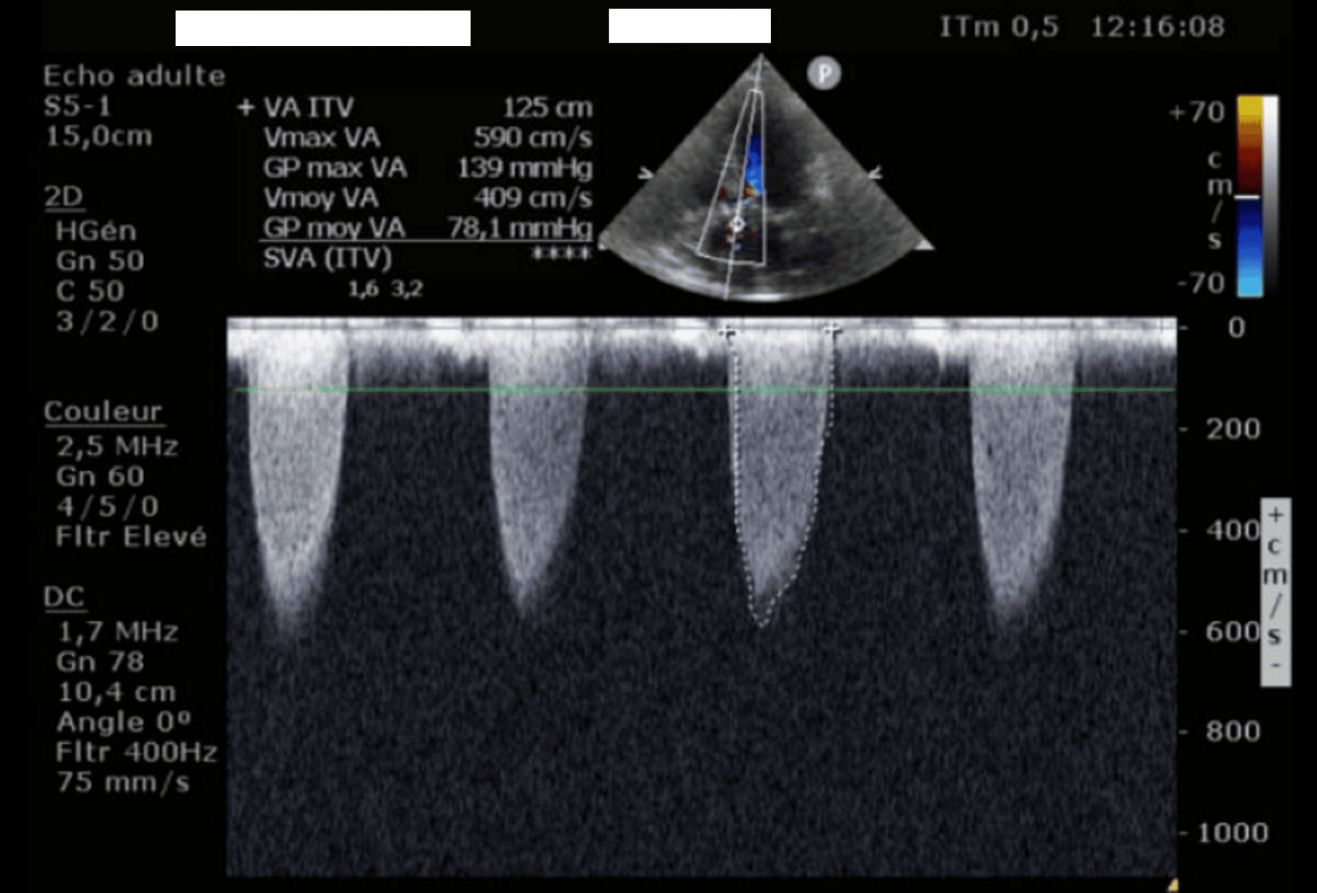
Apical five-chamber view showing a regression in the aortic stenosis parameters
